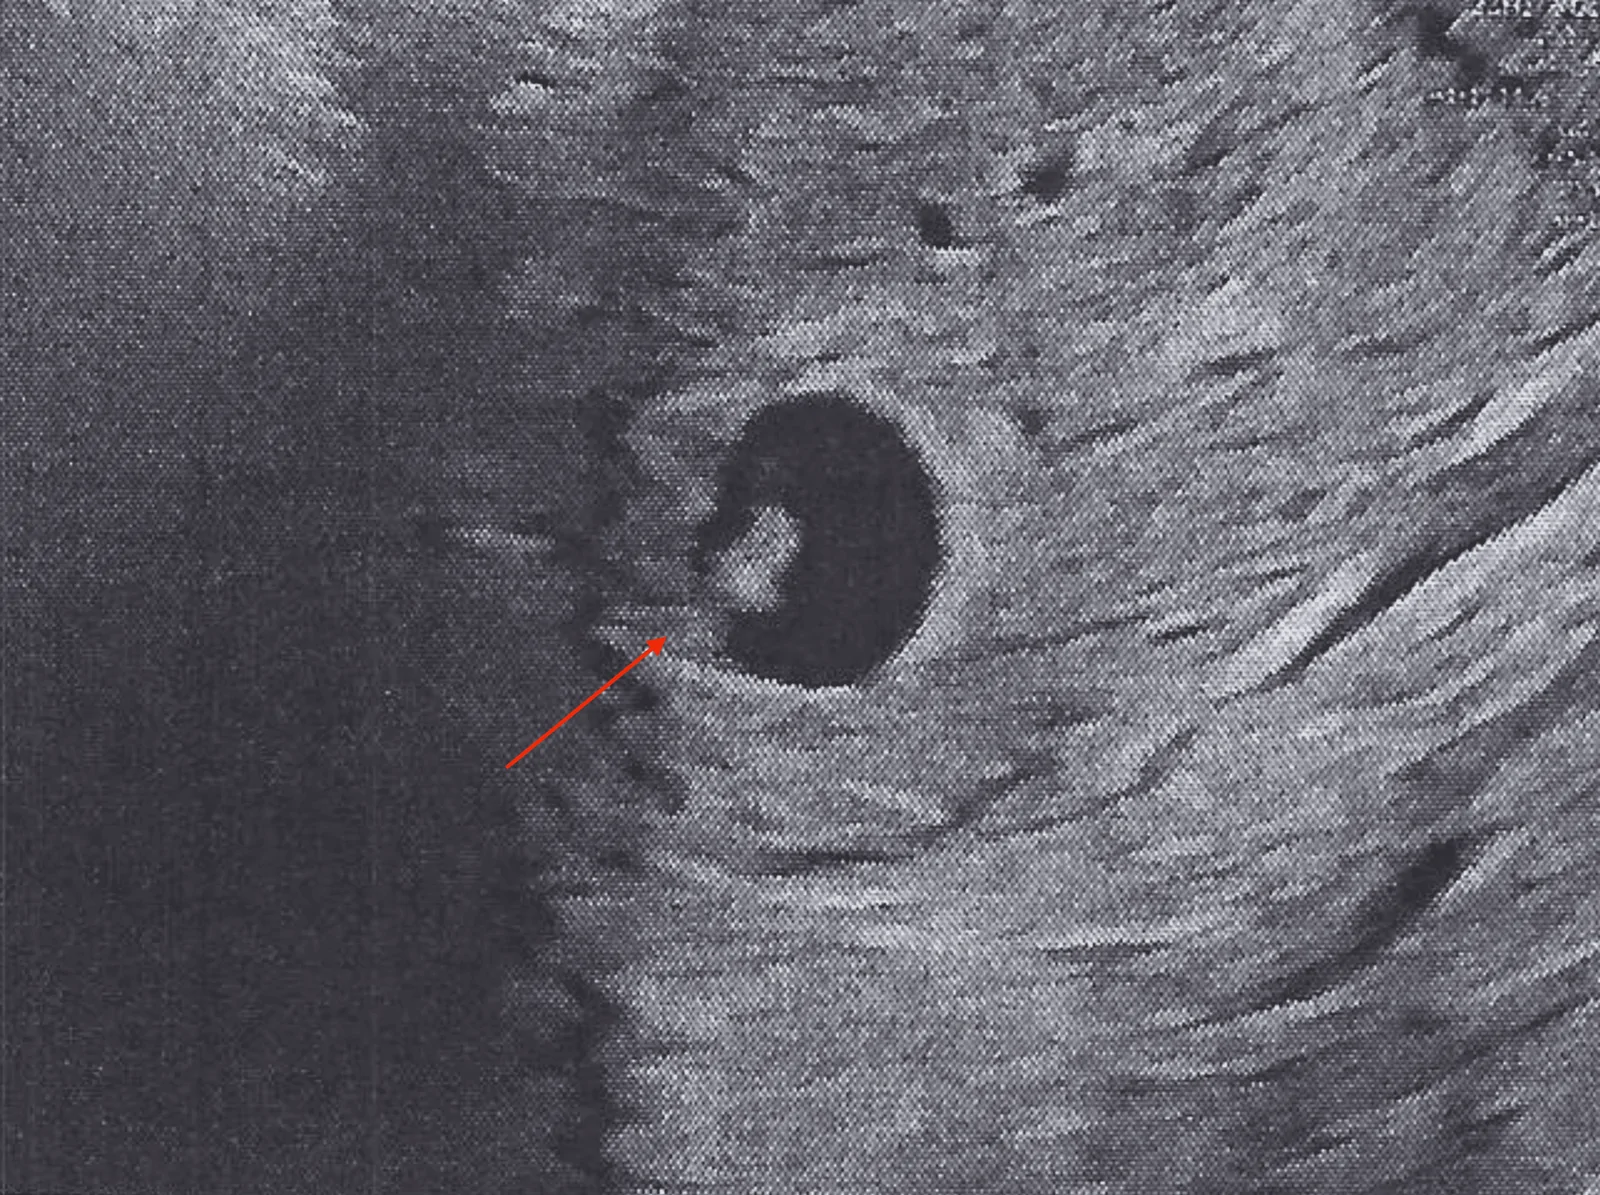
Obstetric ultrasound Obstetric ultrasound with a red arrow demonstrating a gestational age of five weeks and six days.
